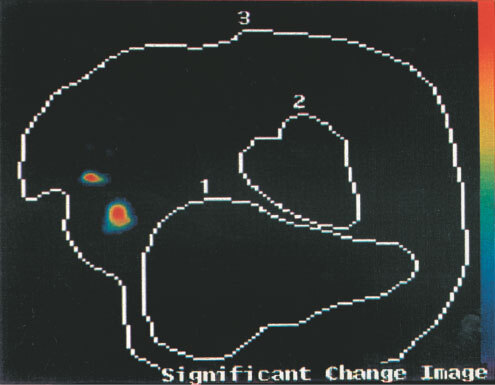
Patient W M 18, Tc-99m hHMFG-1 radioimmunoscintigraphy. Positive right axillary nodes and breast cancer.
